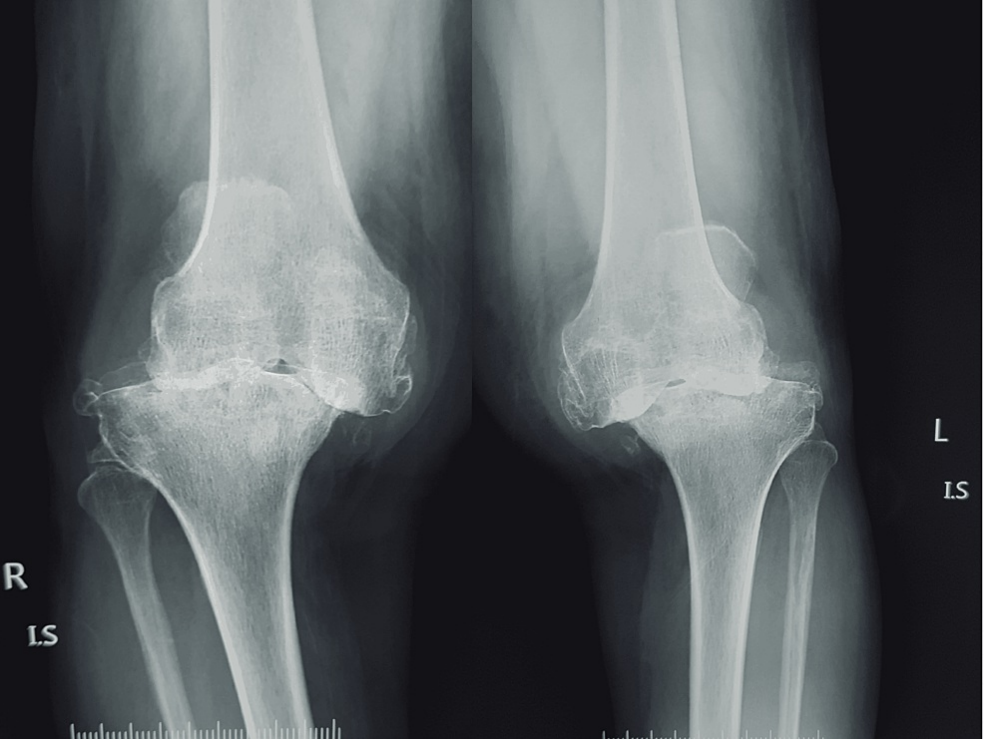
Anteroposterior radiograph of the bilateral knee joint in standing posture with grade IV osteoarthritis with large osteophytes, obliteration of joint space, subluxation of joint, and varus deformity of knees.
